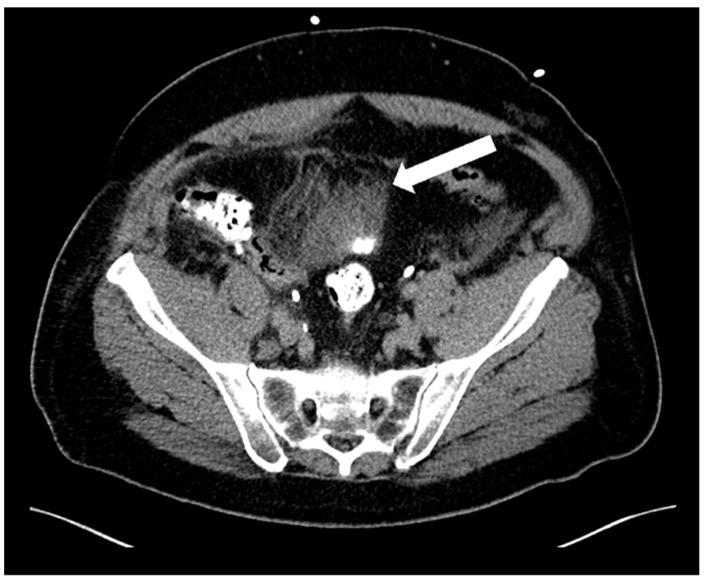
Axial CT image showing a small self-resolving haematoma (arrow) over the bladder dome as a complication of CT-guided drainage.
